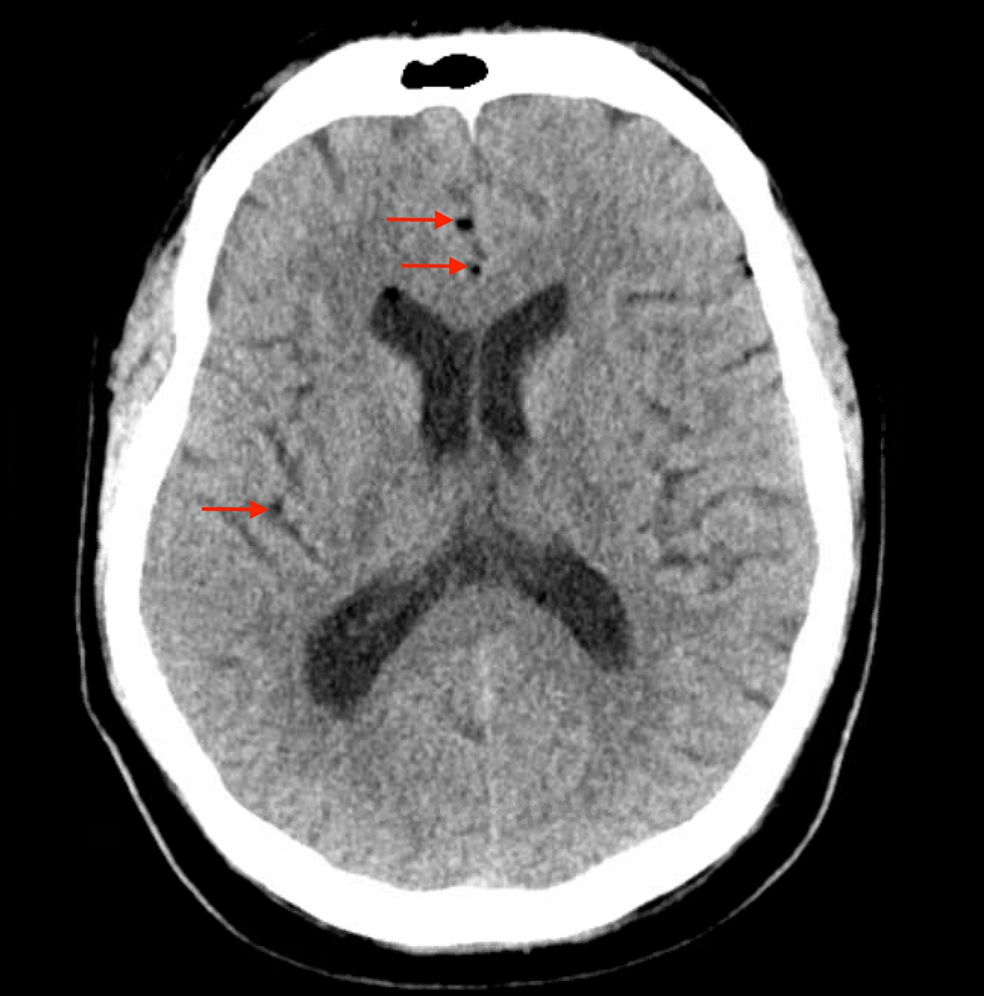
Brain CT scan without contrast, pneumocephalus Red arrows show gas-like foci, pneumocephalus.
